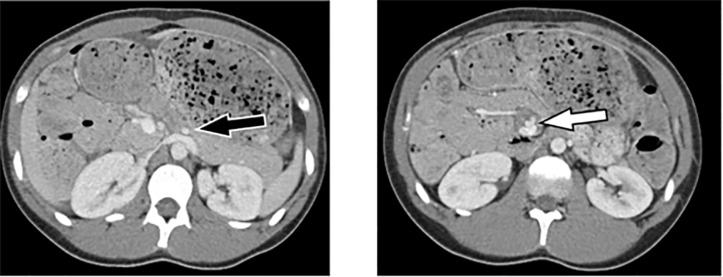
Left-hand image showing branch point of the superior mesenteric artery from the abdominal aorta on axial computed tomography views (black arrow). Right-hand image showing “whirlpool” sign (white arrow).
